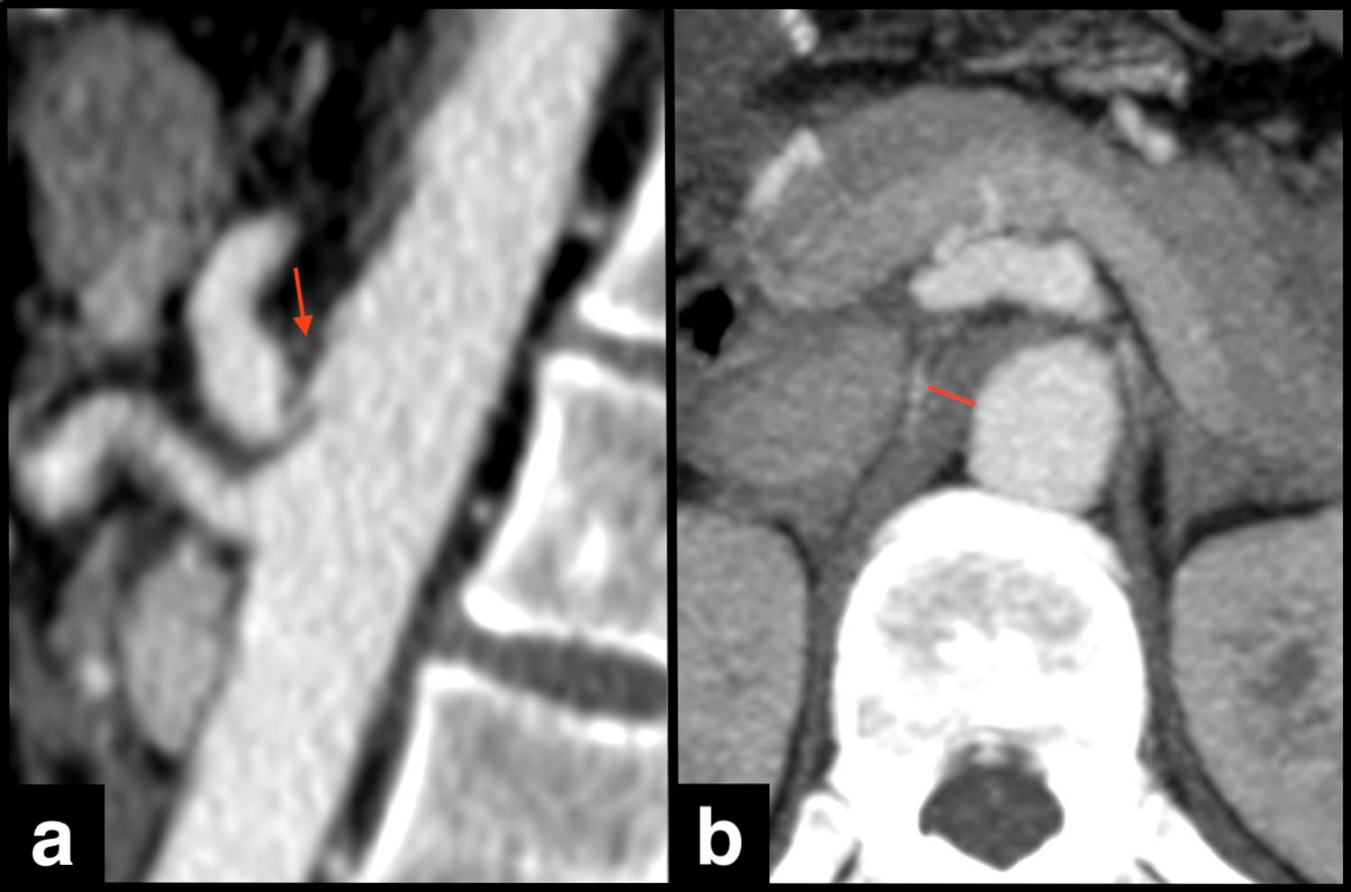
Celiac artery compression by median arcuate ligament. Sagittal (a) and axial (b) CTA images showing the classic hook-shaped configuration (arrow in A) with severe stenosis at the origin of the celiac artery (CA) and post-stenotic dilatation, in the absence of atherosclerotic plaques. The maximum thickness of median arcuate ligament was measured in the axial plane, at the level of the CA origin (b)
